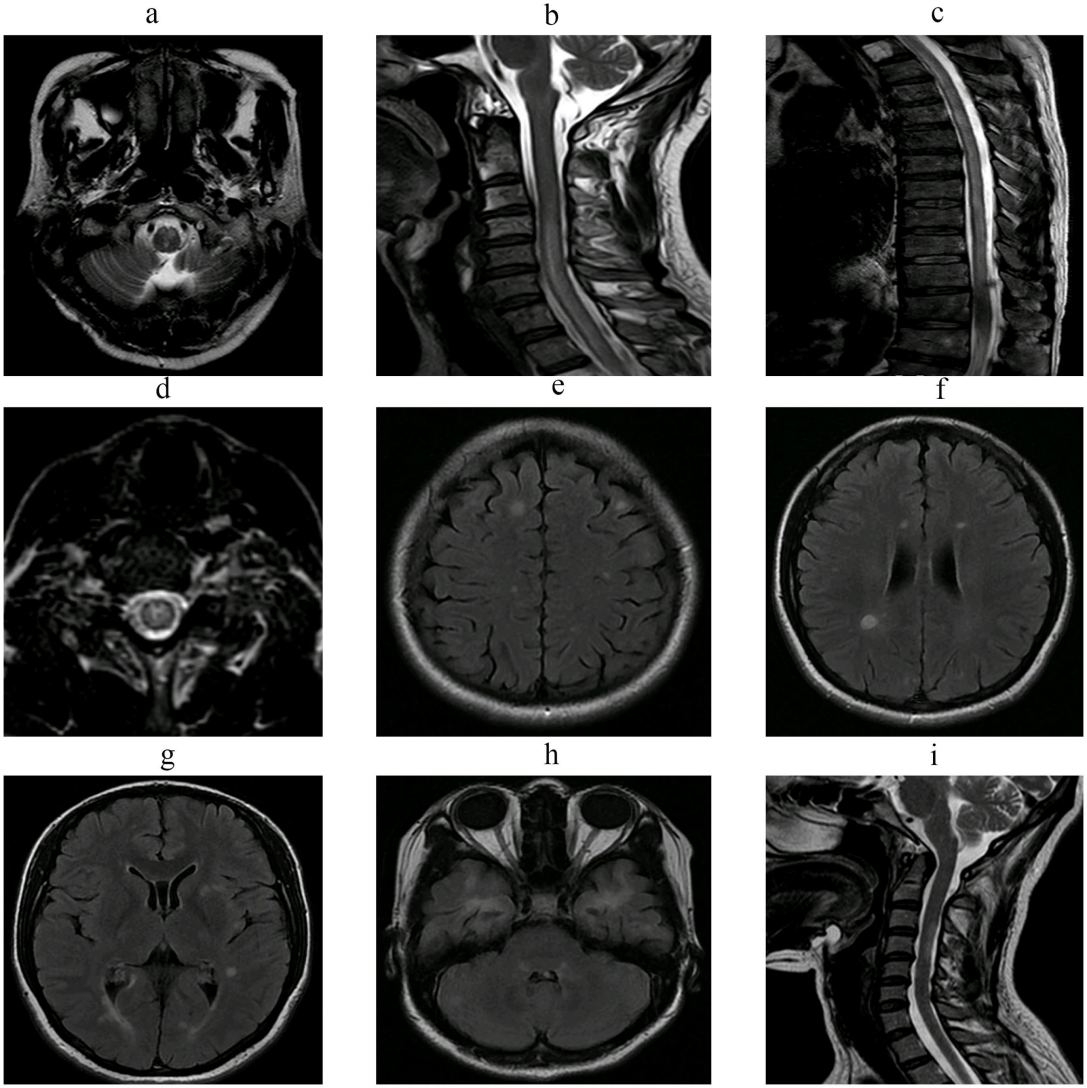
(a–d) Show MRI findings of a 48-year-old patient with NMOSD. In (a), patchy abnormal signals are observed in the medulla, presenting as hyperintensity on T2WI. (b–d) Demonstrate elongated abnormal intramedullary signals extending from C3 to T11, with the lesion located centrally within the spinal cord on axial view and showing slightly increased signal intensity on T2WI. (e–i) Show MRI findings of a 39-year-old patient with MS. (e–h) Display multiple ovoid and nodular abnormal signal lesions in the bilateral cerebral hemispheres, right cerebellar hemisphere, and brainstem, appearing as hyperintensity on T2-FLAIR. (i) Demonstrates patchy abnormal intramedullary signals in the cervical spinal cord with ill-defined margins, presenting as slightly increased signal intensity on T2WI.
